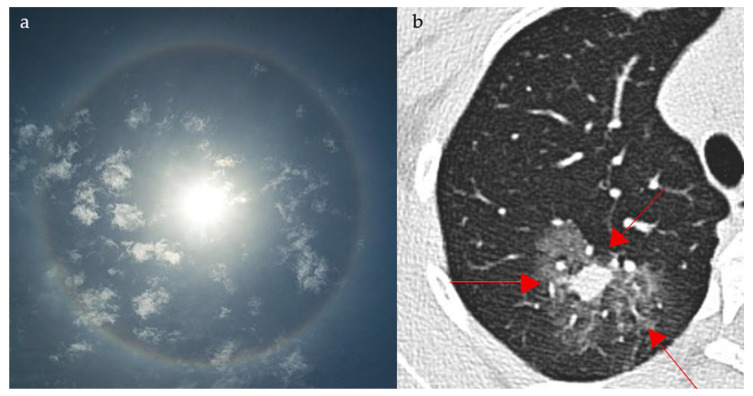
The appearance of a halo surrounding the sun (a), resembling a ground glass opacity surrounding a pulmonary nodule, which represents hemorrhage in a case of early pulmonary aspergillosis on axial CT images of the chest (red arrows) (b).
